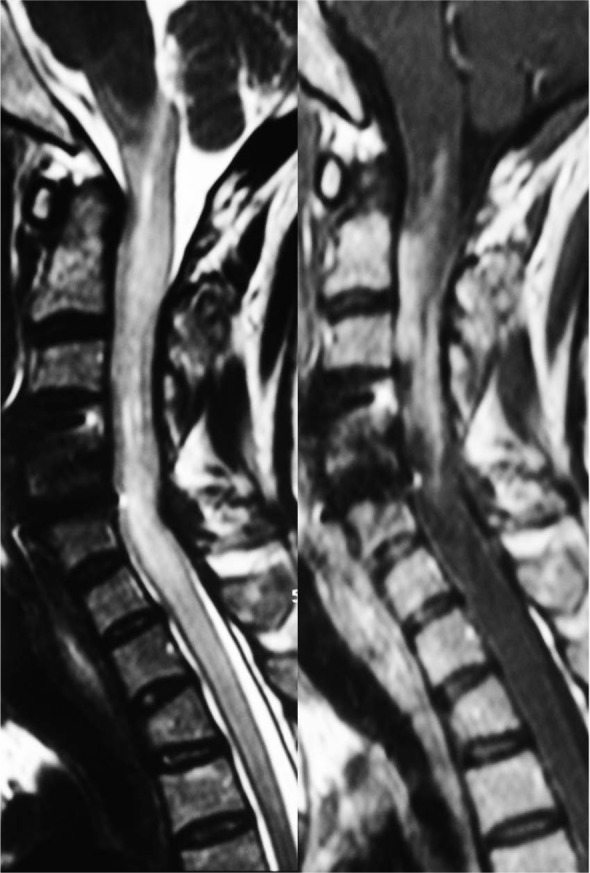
Sagittal T2- (left) and T1-weighted (right) magnetic resonance imaging (MRI) of the cervical spinal cord from case 2 shows a longitudinally extensive, edematous, enhancing, centrally located cervicothoracic cord lesion extending into the medullary region, during the second myelitis attack.
